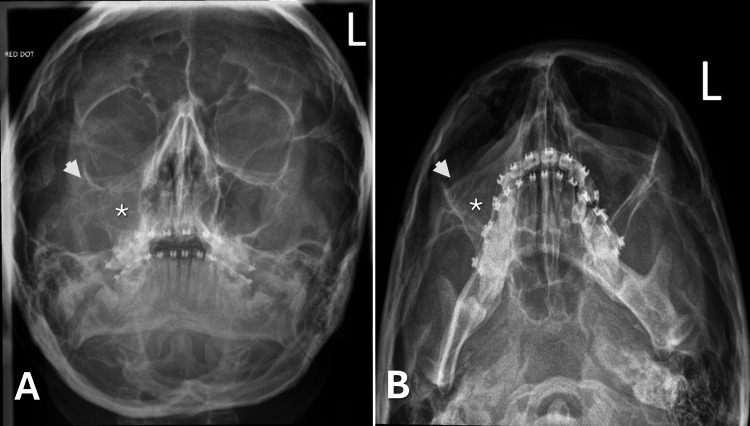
Occipitomental radiographs (A: 0° and B: 30°) demonstrating right maxillary sinus opacification and asymmetry of the right malar contour relative to the left. The asterisk indicates the right maxillary sinus. The arrow indicates the right malar contour.
